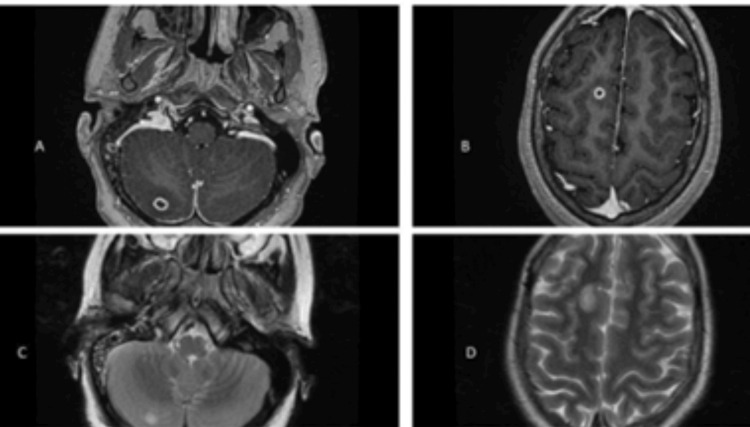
Contrast MRI with MRV brain showing an increase in the size of the right cerebellar and cerebral nodules (A,B), revealing well-defined peripheral ring enhancement (C,D).
